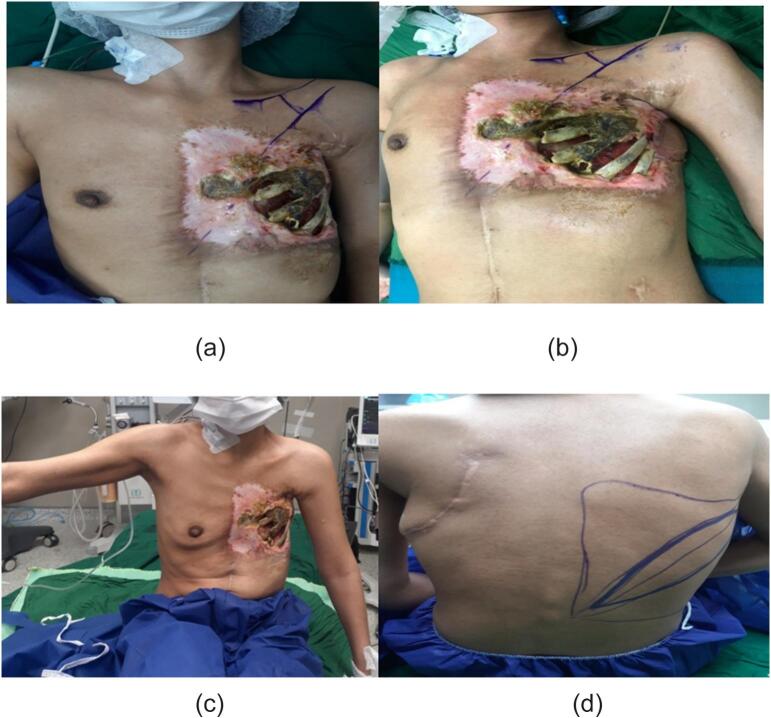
Pre-operative pictures of the patient and thoracoacromial artery direction found by doppler. In c picture restricted arm abduction is apparent.
